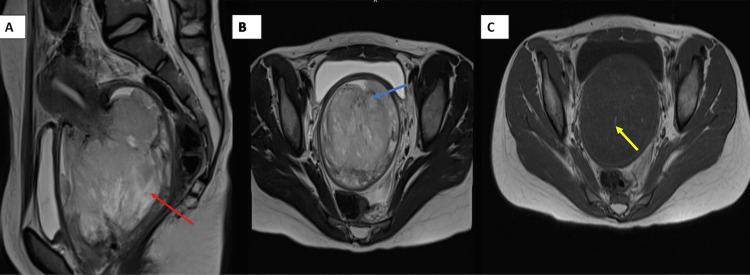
Large exophytic mass occupying the vaginal lumen, arising from the cervix, measuring approximately 86 × 73 mm (axial plane) (A) and 104 mm (sagittal plane) (B), showing heterogeneous high signal intensity on T2-weighted images (A and B) and low signal intensity on T1-weighted images with signs of hemorrhage (C) (A) Axial T2-weighted MRI showing a large exophytic mass arising from the cervix and occupying the vaginal lumen, measuring approximately 86 × 73 mm. The mass displays heterogeneous high signal intensity (red arrow). (B) Sagittal T2-weighted MRI revealing the same mass measuring 104 mm in craniocaudal length, with similar high T2 signal (blue arrow). (C) Axial T1-weighted image demonstrating low signal intensity within the lesion, with focal areas of high signal suggestive of hemorrhage (yellow arrow). MRI: magnetic resonance imaging
